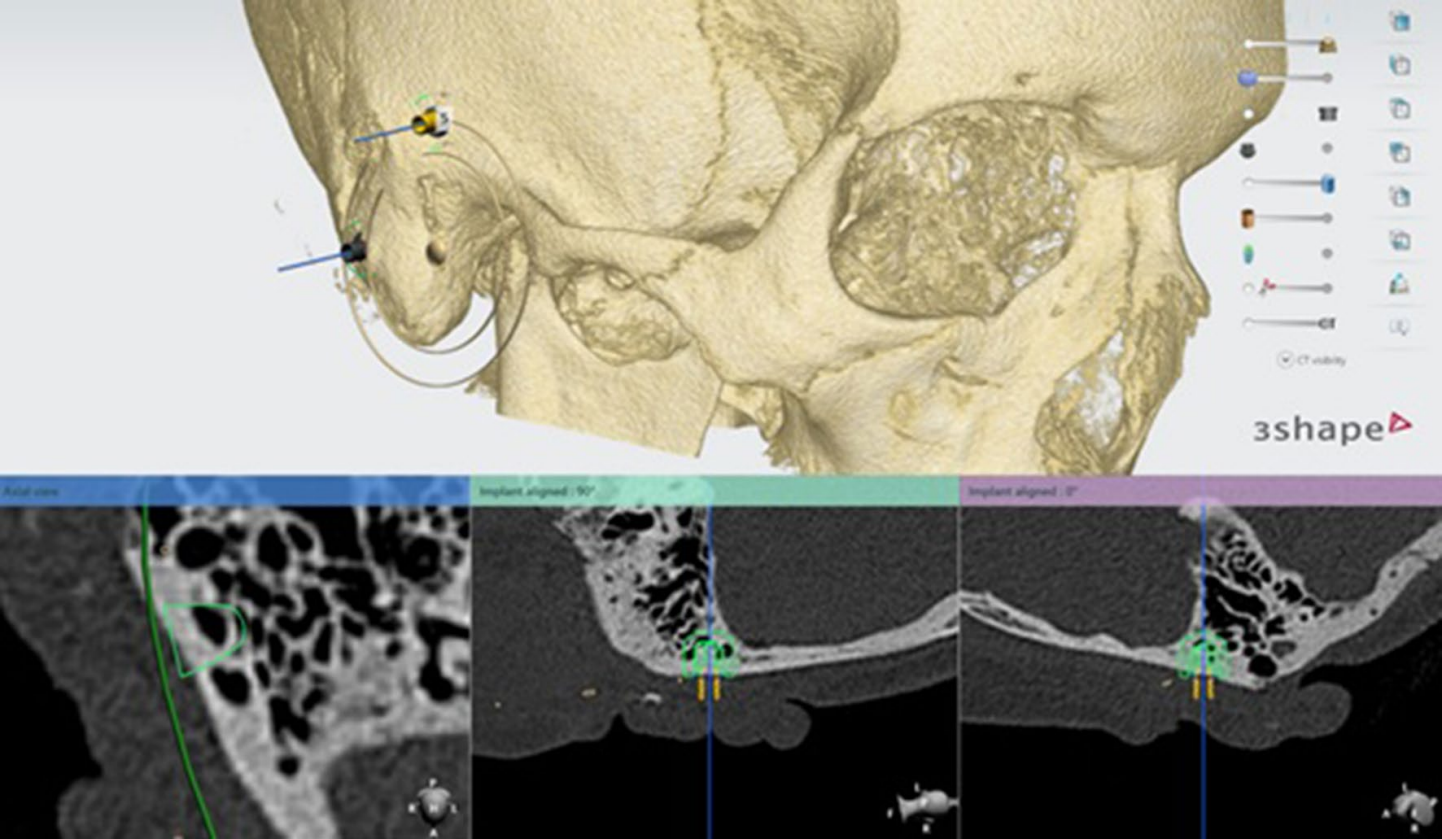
Pre-operative planning of the implants using a CT-scan
